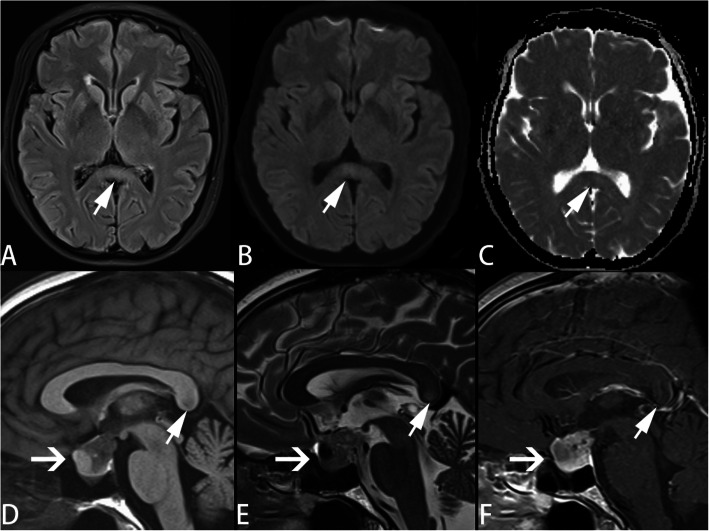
The MRI finding of case 5. The arrow (➚) showed lesion center on SCC characterized by hypo-intensity on T1-FLAIR (D), hyper-intensity on T2WI (E), T2-FLAIR (A) and DWI (B) and low ADC value (C). Signal of lesion center on SCC showed no enhancement (F). The lesions of SCC were resolved at telephone follow-up 15 days later. Moreover, there was a large oval-shaped lesion in the sellar region with a size of about 18 × 19 × 24mm (D & E; ➜). The abnormal signal was mixed. The lesion showed uneven enhancement (F). Pathological diagnosis confirmed pituitary adenoma
